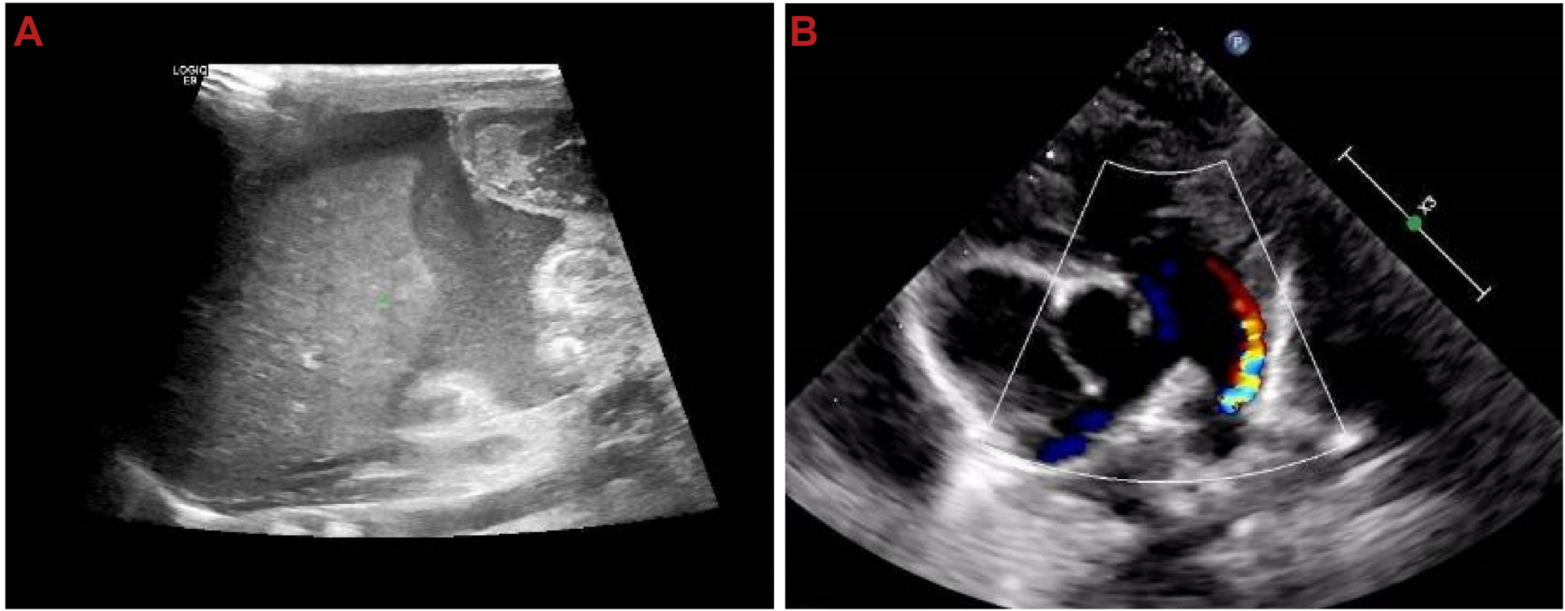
Ultrasound shows fluid-filled hypoechoic areas around the liver and spleen, as well as cardiac structural and functional abnormalities. (A) Hypoechoic fluid-filled areas around the liver and spleen; (B) neonatal echocardiography showing cardiac structural and functional abnormalities.
